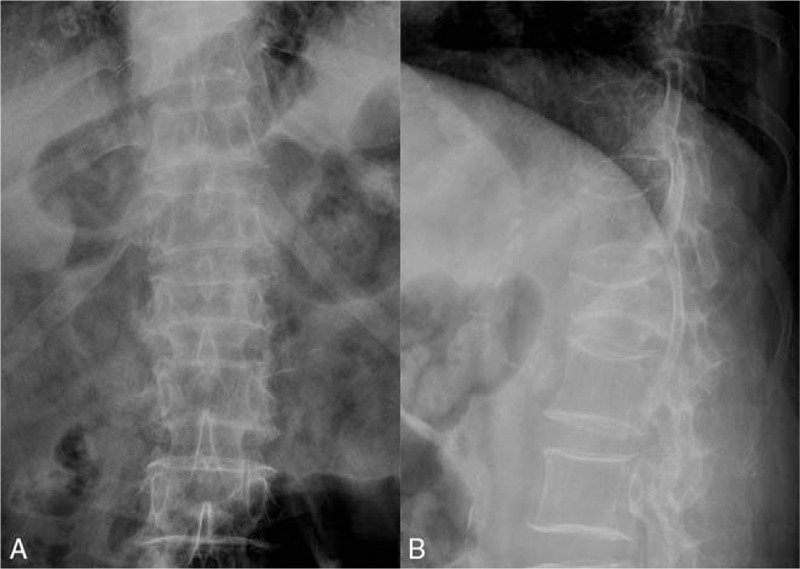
Preoperative X-rays of the lumbar. (A) Anterior-posterior and (B) lateral radiographs showed L1 vertebral compression fractures.
